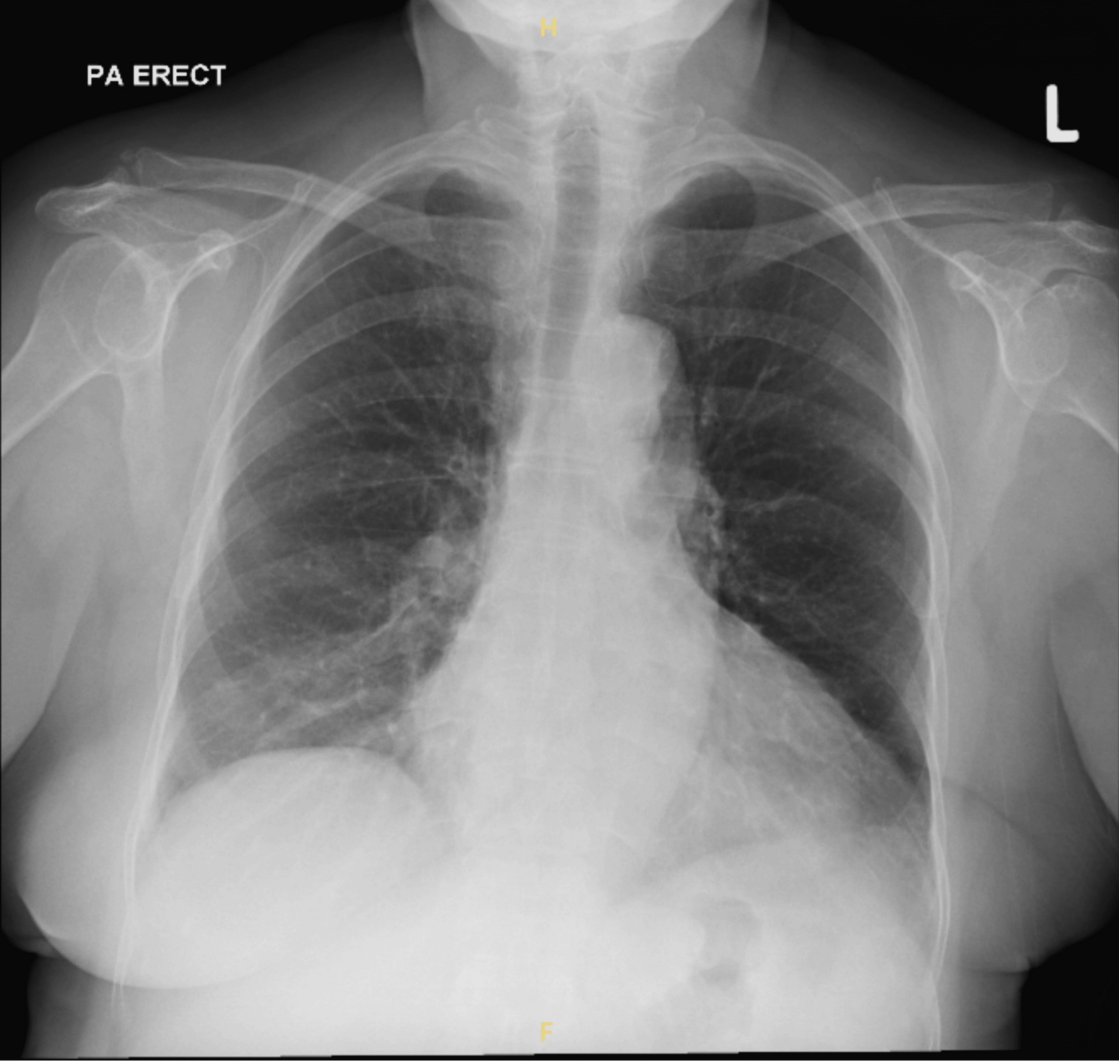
A follow-up chest radiograph at two weeks postoperatively showing no residual or recurrent mediastinal mass
